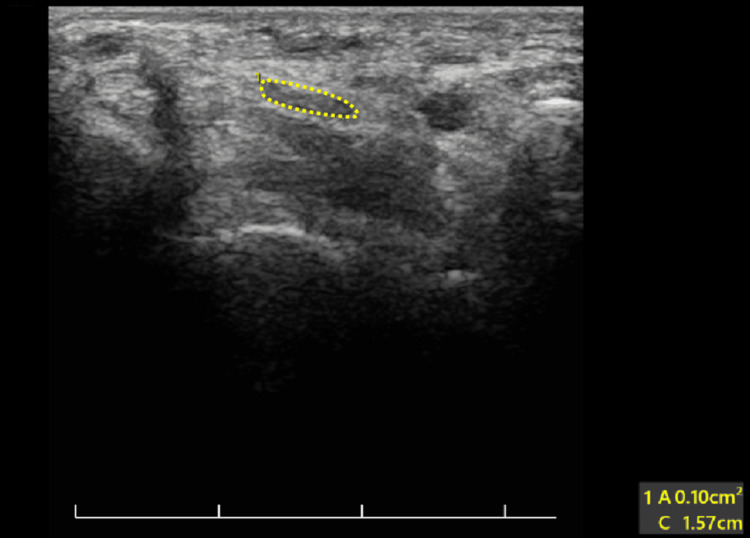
USG showing a cross-section of the median nerve in the carpal tunnel of the left hand of a low user (dotted yellow lines) USG: ultrasonography
